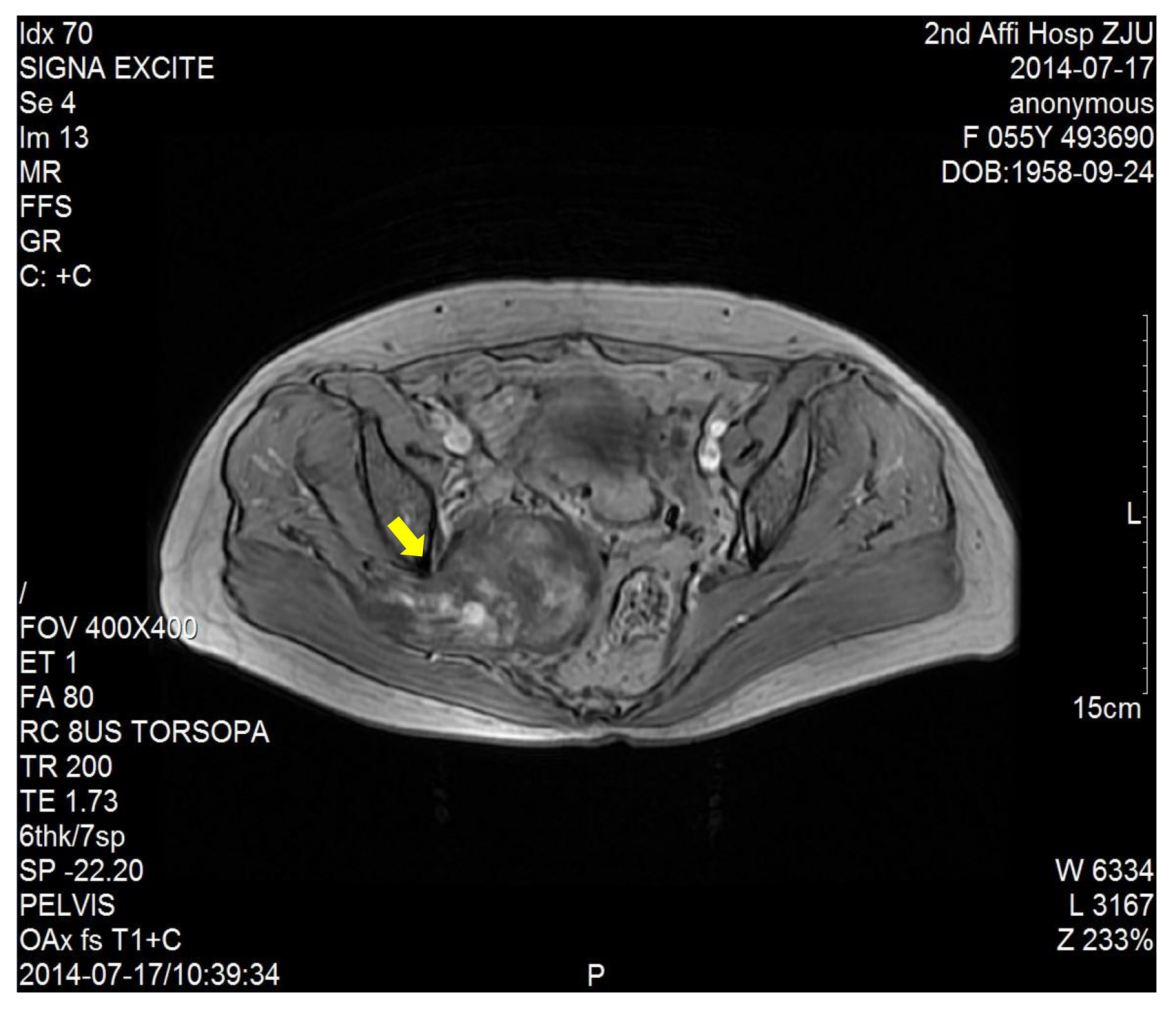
T1-weighted image showed a right sacral mass as a dumbbell-shaped configuration and mixed high signal intensity on the basis of low signal intensity
